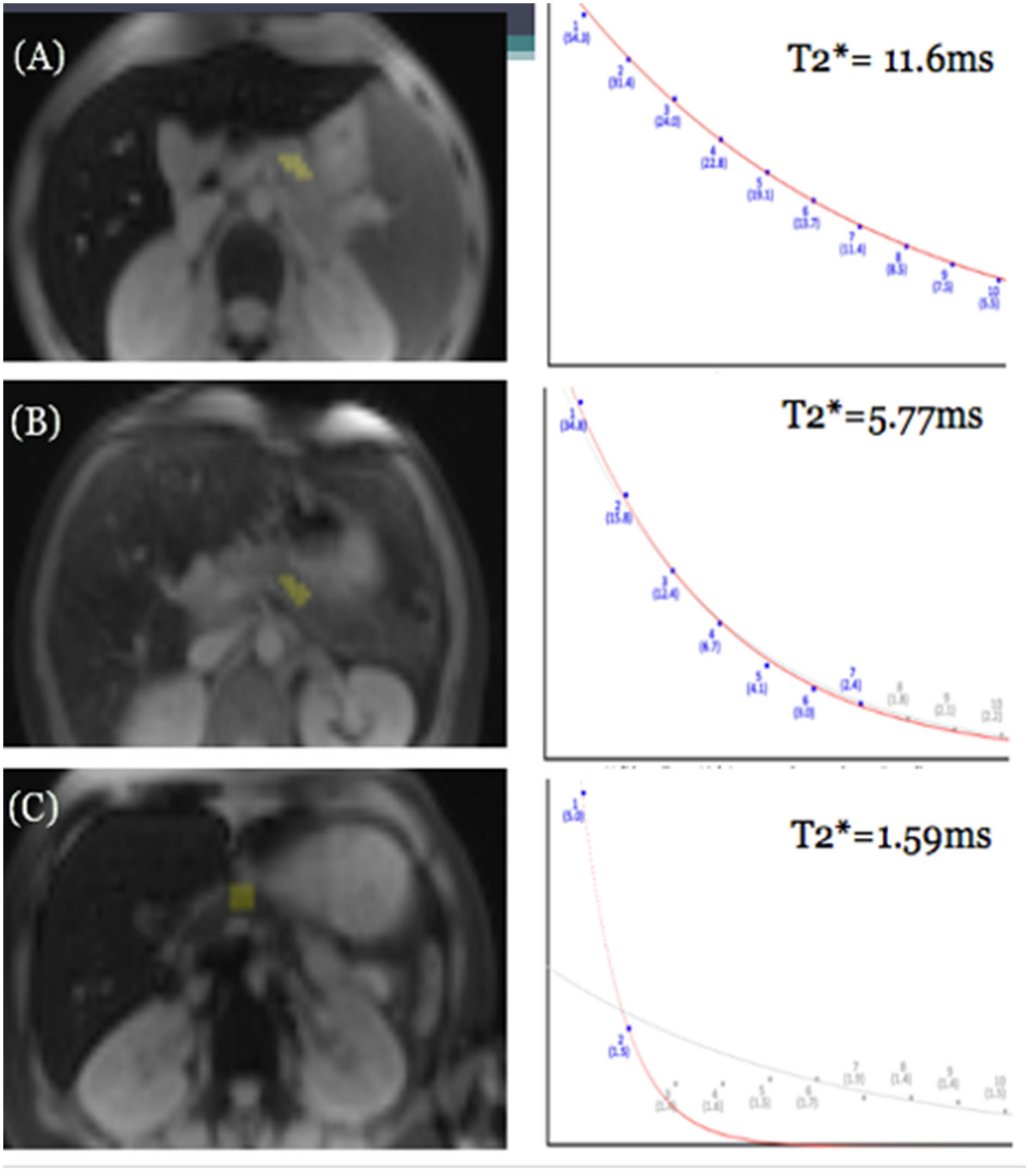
GRE MRI images of three different patients showing mild (A), moderate (B), and severe (C) iron deposition in the pancreas at the level of the splenic vein, and corresponding differences in rate of signal decay resulting in different pancreatic T2* values in patients with mild, moderate, and severe pancreatic iron overload.
